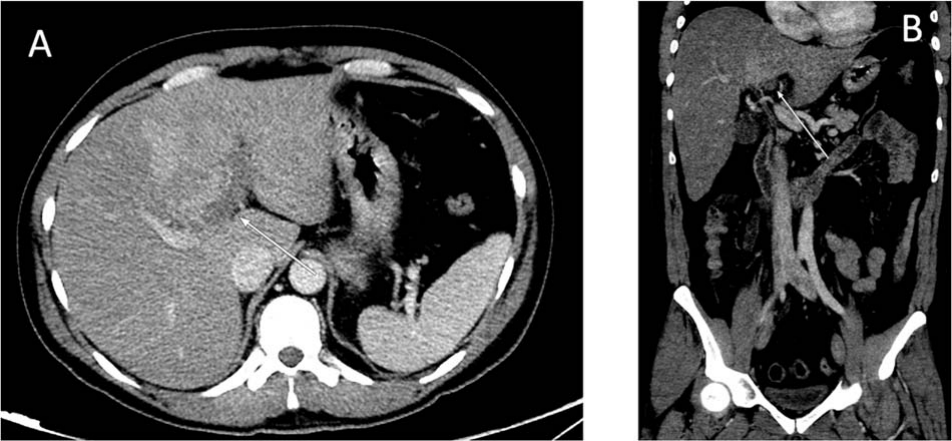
Contrast-enhanced CT scan of the abdomen: (A) axial cut; (B) coronal cut. Arrow indicates left portal vein thrombosis.
